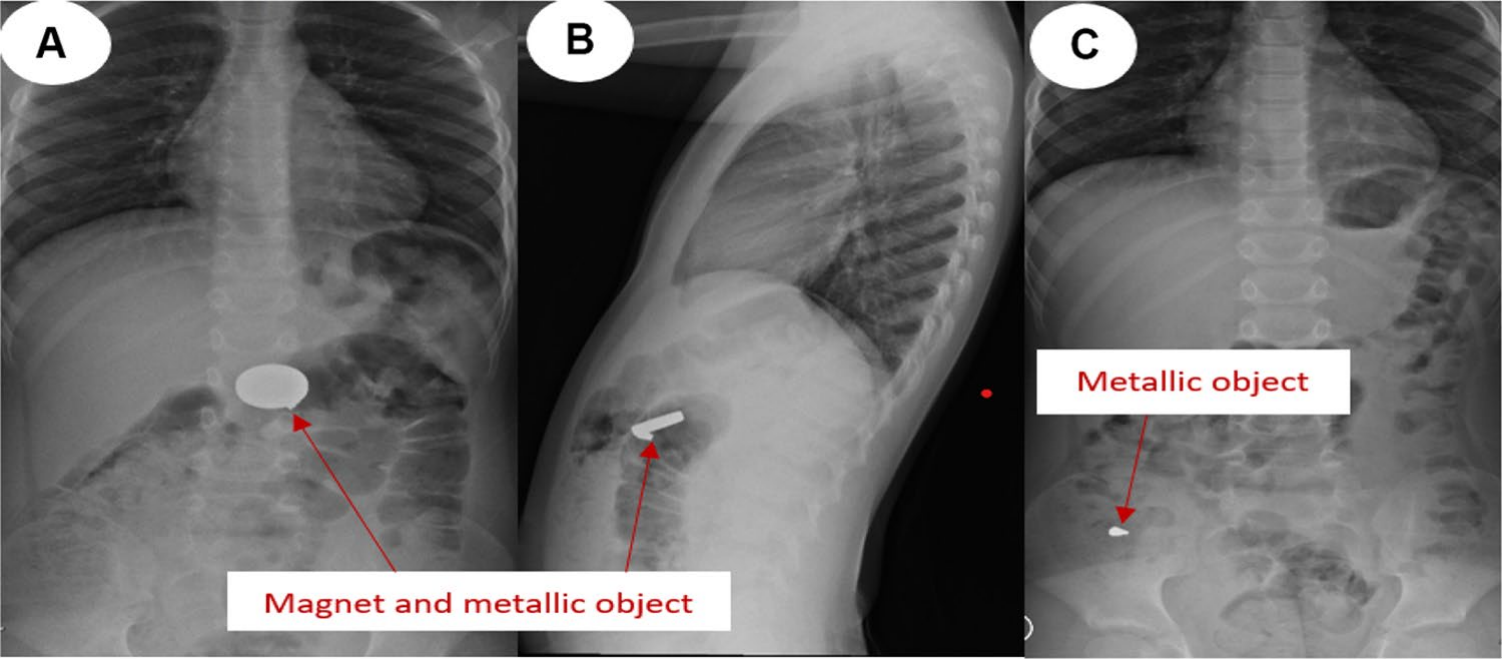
Radiograph showing magnets and metallic objects. Magnet extracted endoscopically and metallic object passed spontaneously.
